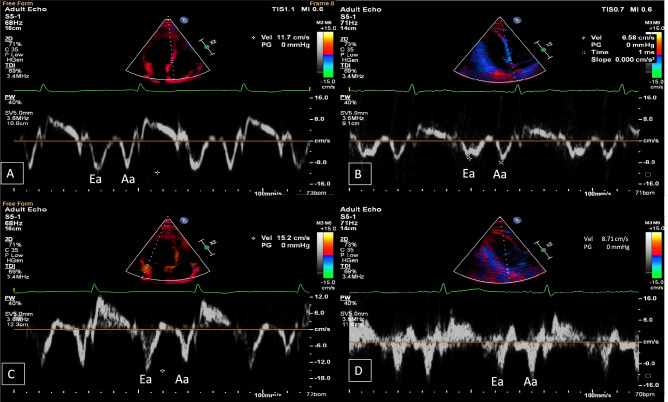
High and low early (Ea) and late diastolic mitral annular velocity (Aa) at the interventricular septal annulus (A, B) and lateral side of the mitral annulus (C, D) measured by tissue Doppler echocardiography
